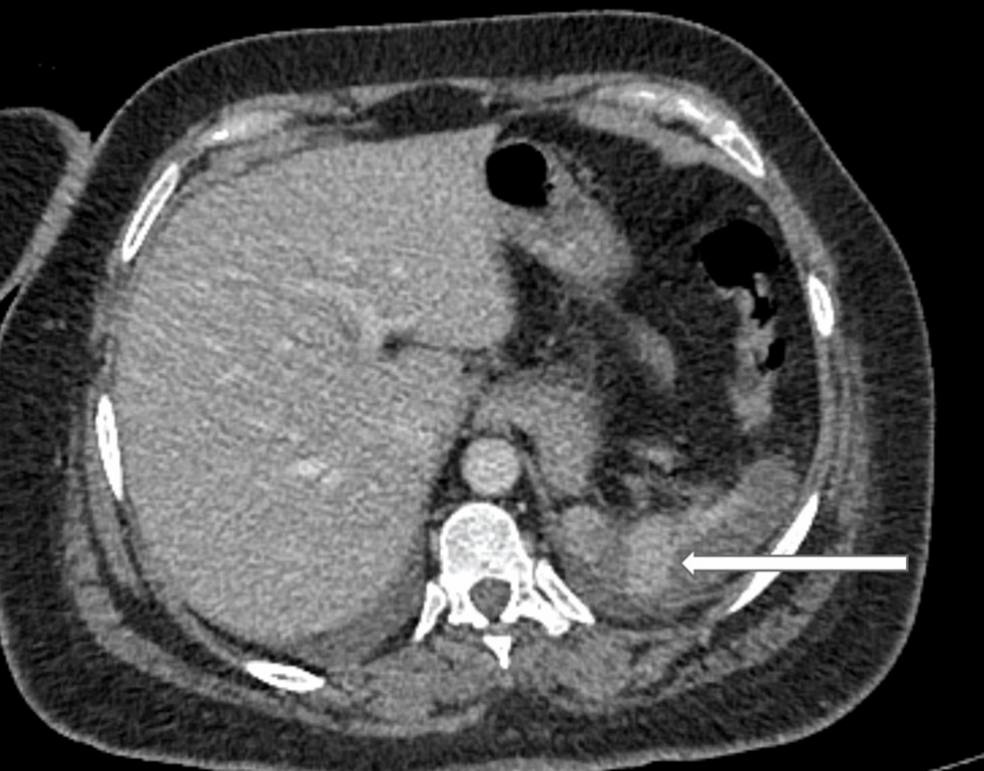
Abdominal CTA (Axial view) showing the splenic infarction (white arrow).
